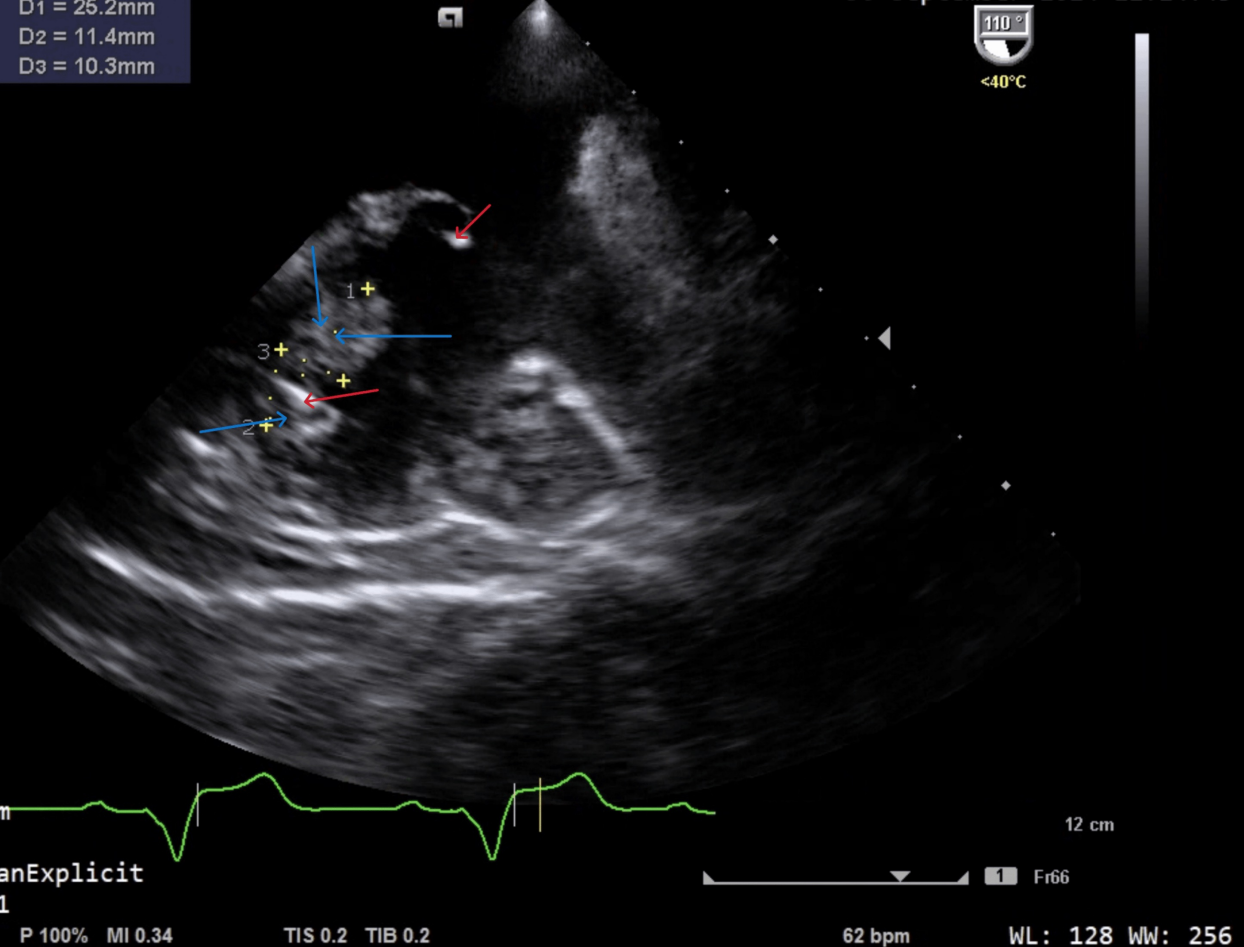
Initial echocardiography findings. Multiple hypermobile vegetations visualized on the pacemaker lead, prolapsing from the right atrium into the right ventricle. Red arrows: pacemaker leads, Blue arrows: infected masses
